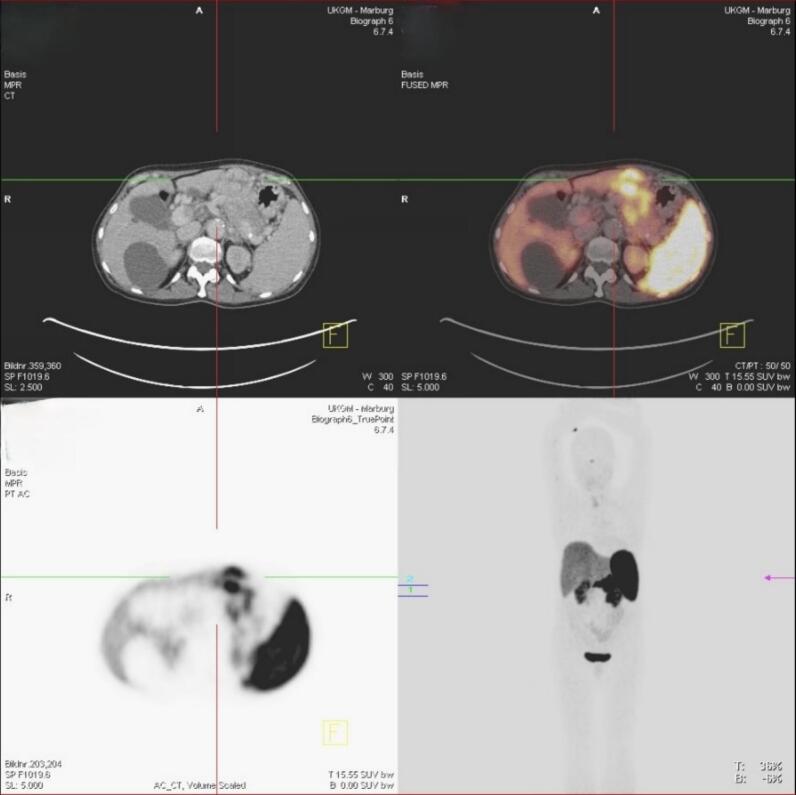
Ga 68-DOTATOC PET-CT: tracer positive tumor in the corpus/tail area of the pancreas with positive lymph nodes.
